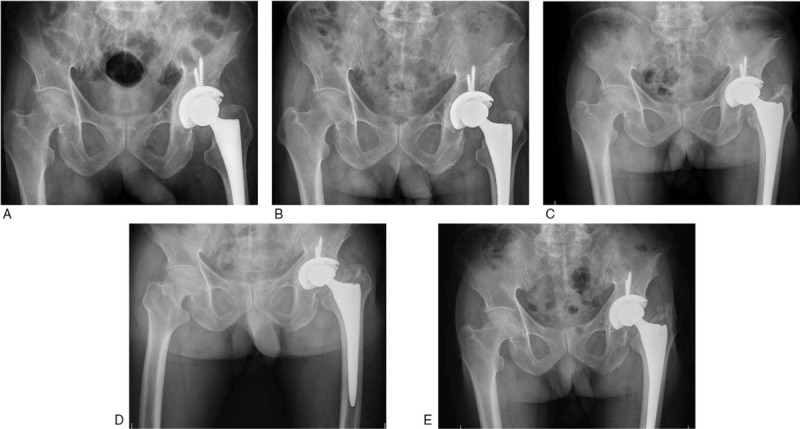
Postoperative follow-up through CR of plain pelvic films. Postoperative follow-up through CR of plain pelvic films. A–E showing pelvic radiographs at 1, 3, 12, 20, and 32 months after surgery, respectively. The left hip prosthesis with good healing of the bone graft without any complication.
